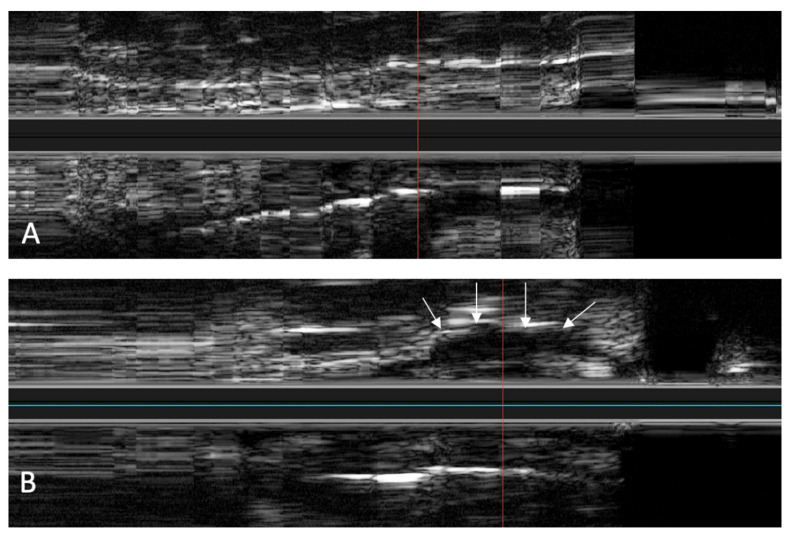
In vivo longitudinal ultrasound image of the ET before (A) and after (B) placement of an HA depot. In (B), the HA depot is anechogenic next to the tubal lumen (marked by the white arrows).
